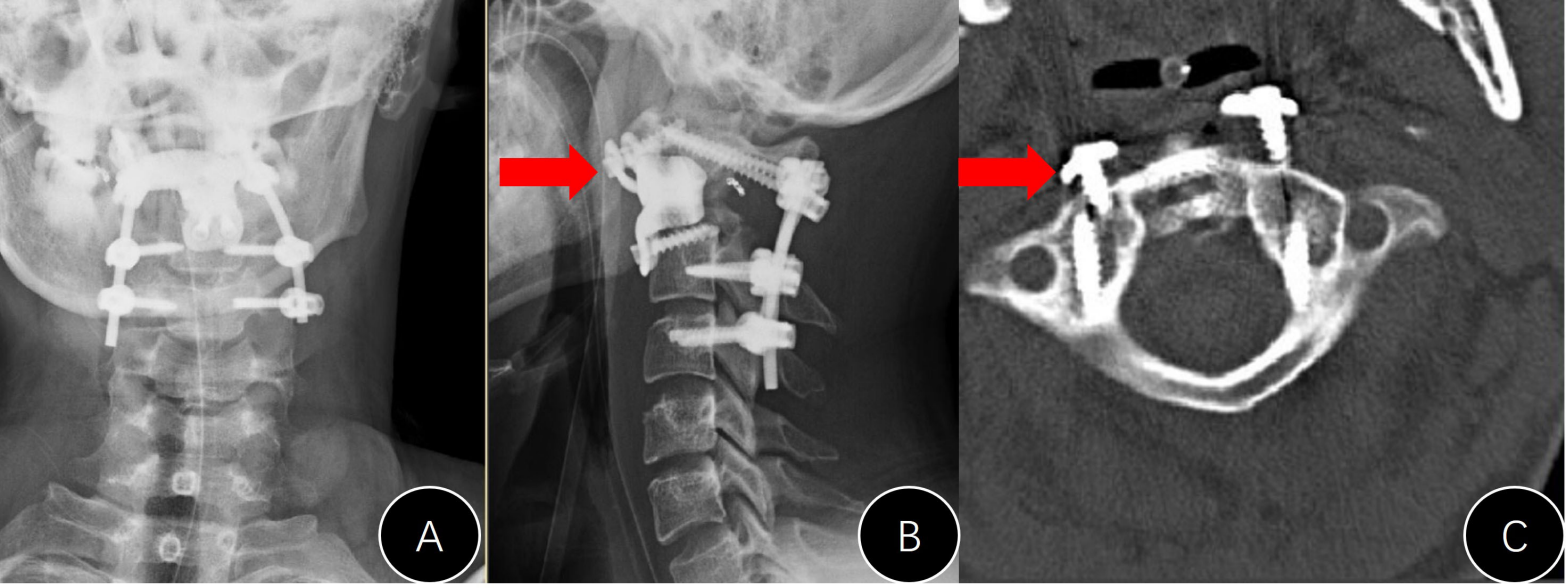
Presentation of case #B7. (A, B) Postoperative x-rays (unsatisfactory cranial attachment to C1 anterior arch); (C) CT film revealed that the cranial screws were not completely nailed in.
